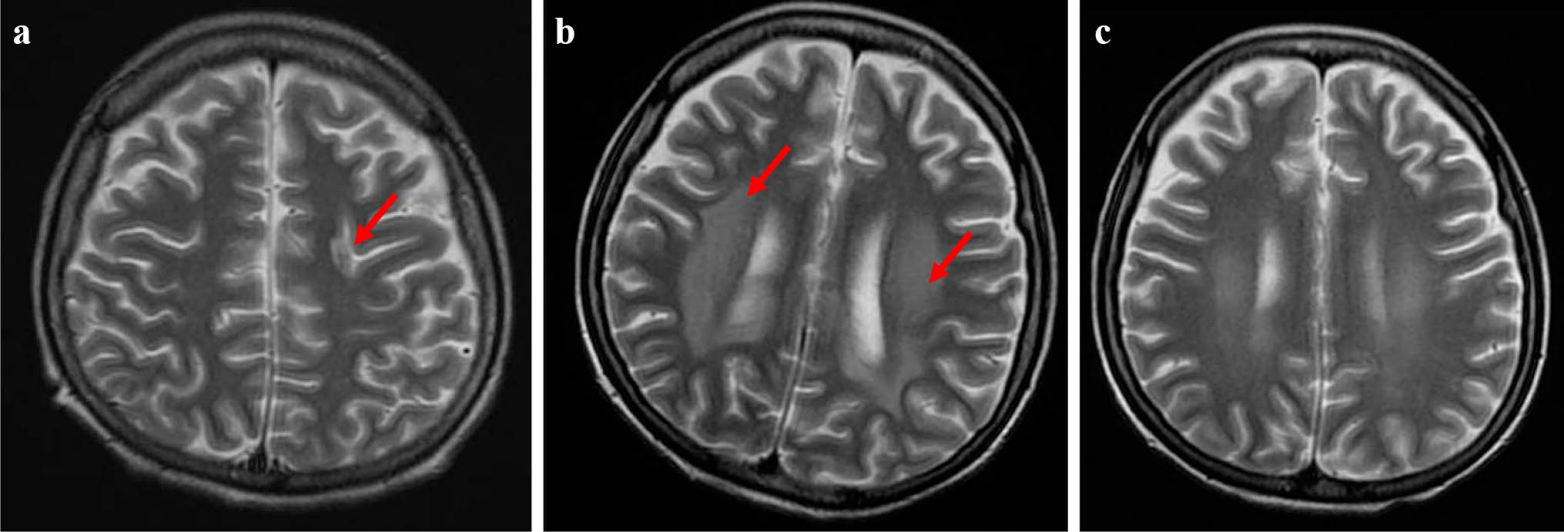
T2-weighted images on head MRI. a A round, high-intensity signal (arrow) was recognized in the white matter in the left parieto-occipital area on the 78th day after the start of chemotherapy and she was admitted to our hospital. b High-intensity signals (arrows) within the cerebral white matter are observed at both sides of the occipital lobe on the 9th hospital day. c After the chemotherapy, this lesion gradually disappeared. This MRI image was obtained on the 20th hospital day
